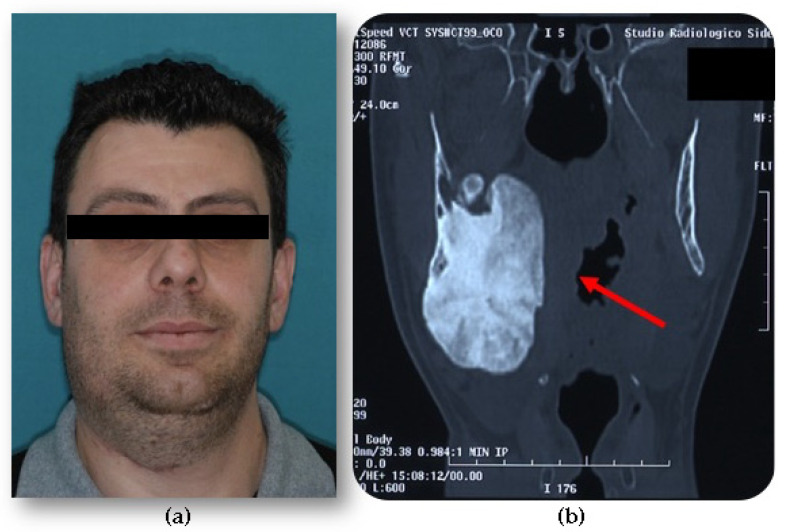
(a) A young male affected by an extensive right mandibular osteoma, causing facial swelling. (b) Computed tomography (CT) scan shows diffuse enlargement of the entire right mandible, extending to the lateral and medial sides and causing deformation of the pharyngeal walls.
